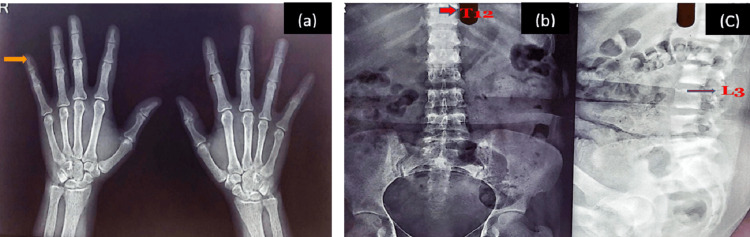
X-ray bilateral hand and an X-ray spine (thoracolumbar region) (a) Bilateral hand X-ray revealing resorption of terminal phalanges and osteopenia, (b) X-ray of the spine revealing concave appearance of T12, and (c) X-ray spine lateral view showing irregular upper and lower surface of L3.
